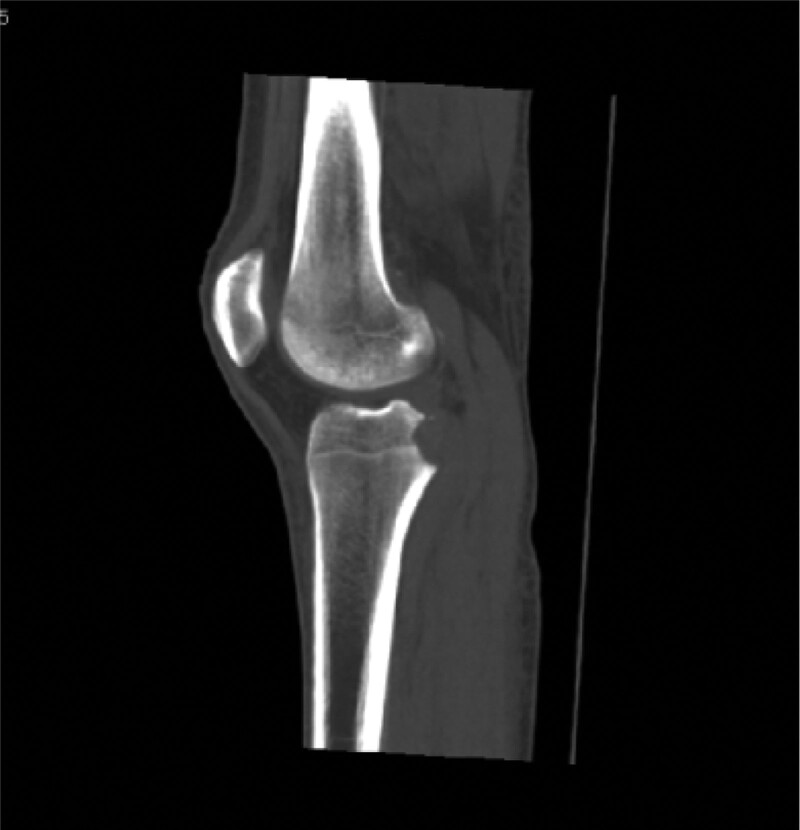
Postoperative CT image in mid-sagittal plane. CT = computed tomography.
